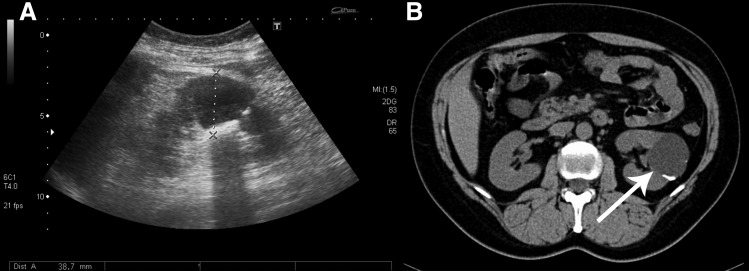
Complicated cyst diameter 41 mm, Bosniak IIF, calcifications in the wall by ultrasonography (A), diagnosed 10 years ago by noncontrast CT (B), no signs of progression.
